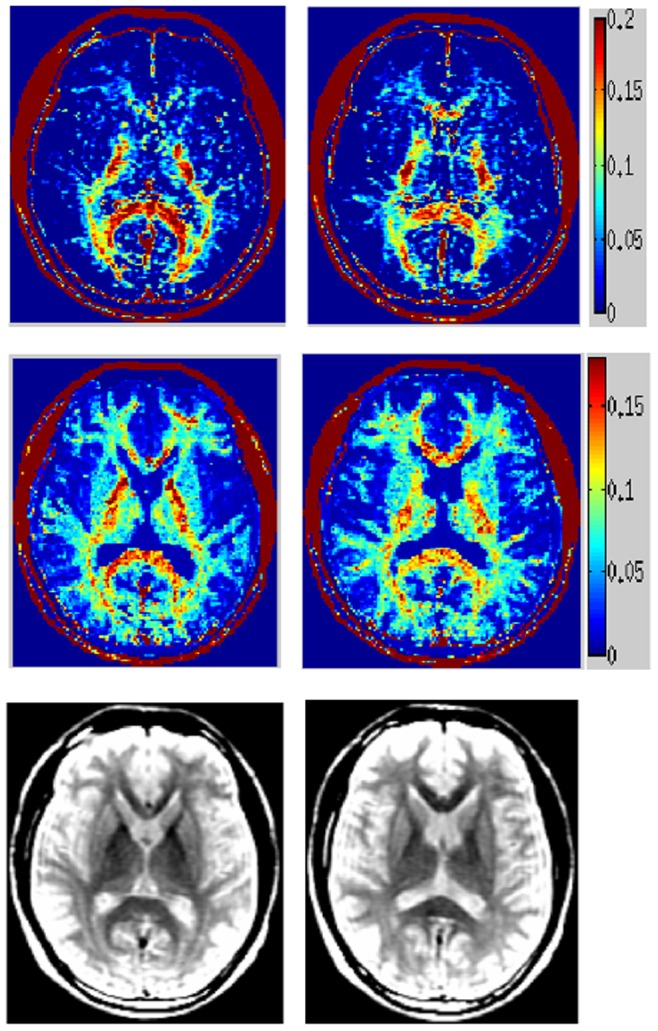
MWF maps of healthy MESE scans acquired at 1.5T. MWF computed from healthy *in vivo* anisotropic MESE scans acquired at 1.5T. Conventional reconstruction (top), spatial constrained reconstruction (middle) and anatomical T2 FLAIR images (bottom). Two consecutive axial slices at the level of thalamus and putamen are shown.
